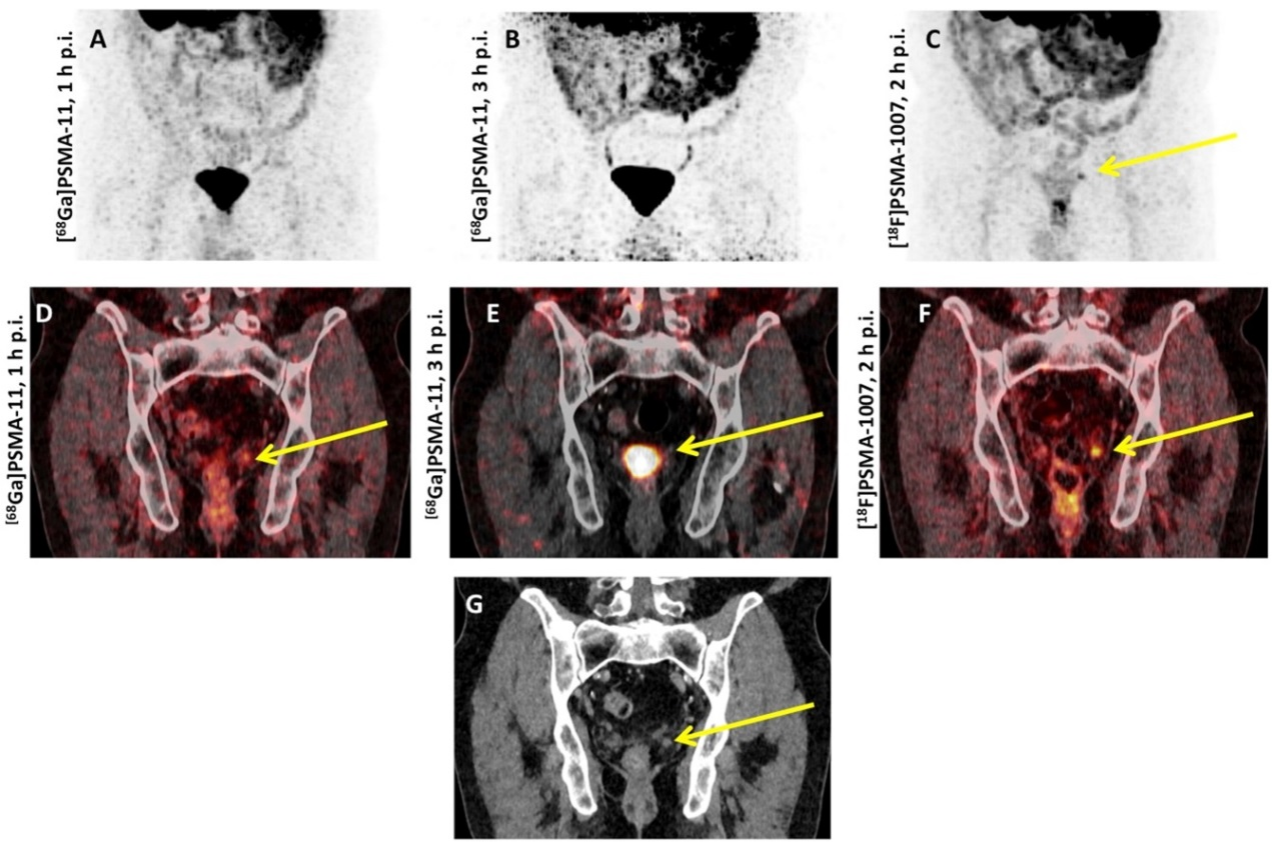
Head-to-head comparison of [^68^Ga]PSMA-11 vs. [^18^F]PSMA-1007 PET/CT. Biochemical relapse after prostatectomy, localized in the fossa of the seminal vesicle on the left. (A,D) early and (B,E) late [^68^Ga]PSMA-11 PET/CT scans which showed an equivocal finding. The residual activity of [^68^Ga]PSMA-11 3 hours p.i. was too low for a final interpretation (E). The additionally performed [^18^F]PSMA-1007 revealed the relapse with a PSMA overexpression, demonstrated on the maximal intensity projection (C) and on the coronal tomogram (F). The relapse showed a correlate on the low-dose CT (G).
